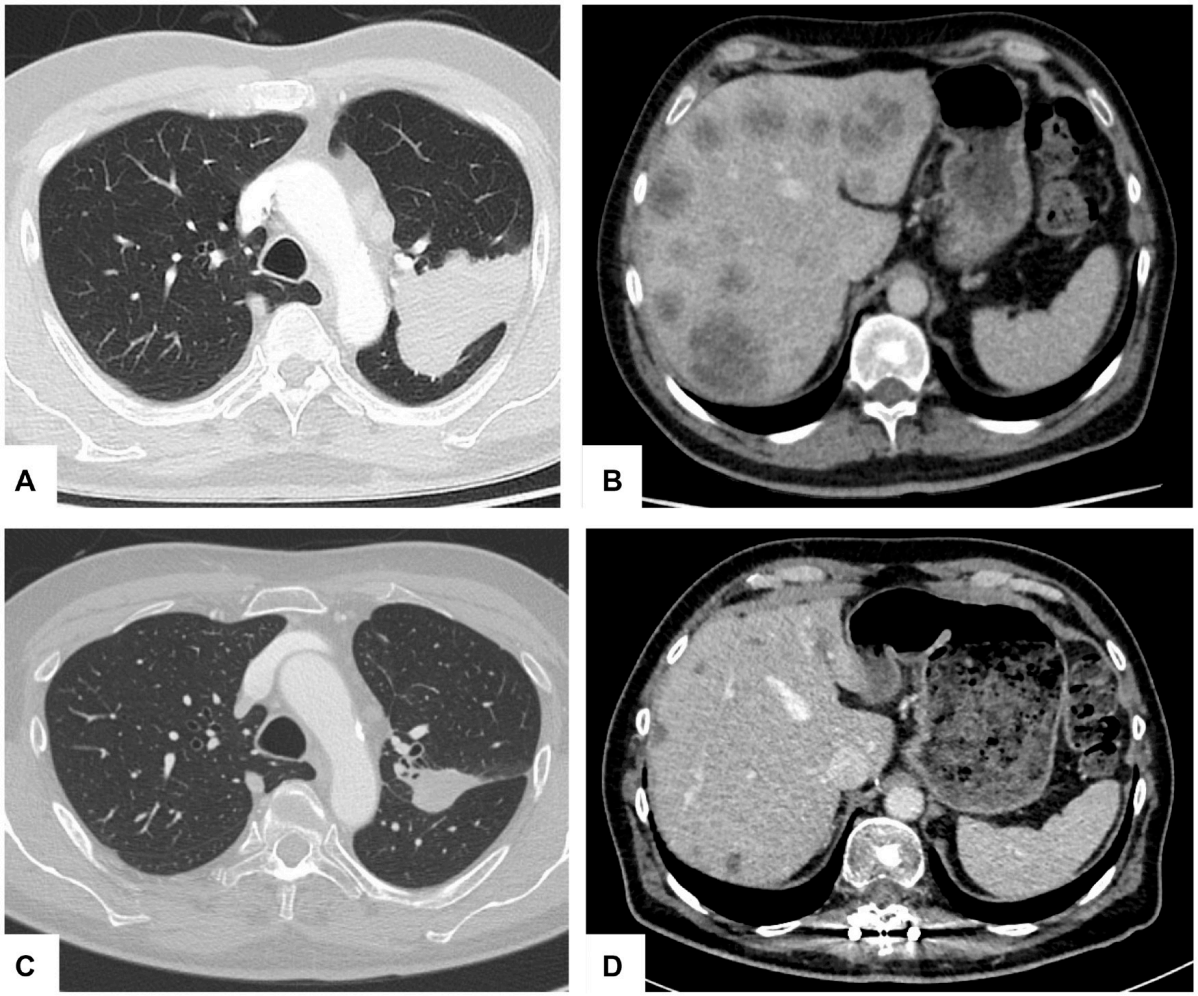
Hepatic and pulmonary tumor response during therapy. Axial computed tomography (CT) scans of left upper lobe pulmonary tumor and hepatic metastatic lesions at initial diagnosis (A, B) and after alectinib therapy ten months later (C, D) showing partial remission (PR).
